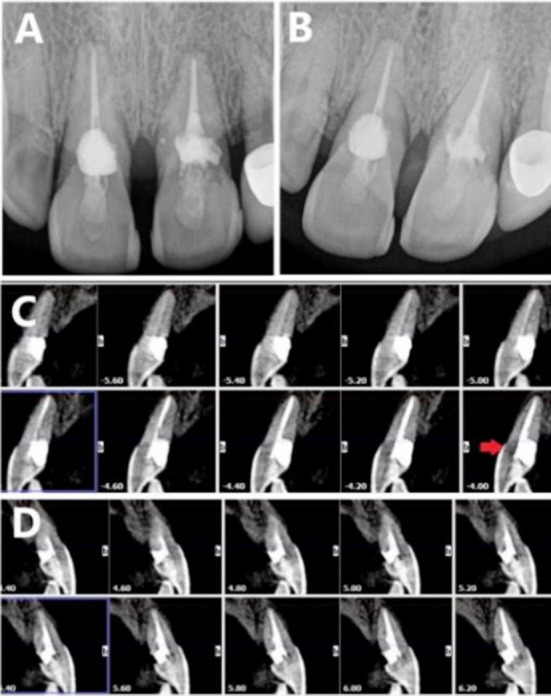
Follow-up radiographs after six years (A) Straight PAR, (B) Distally shifted PAR, (C) CBCT sagittal sections #11, red arrow showing new resorptive activity, (D) CBCT sagittal sections of #21.
